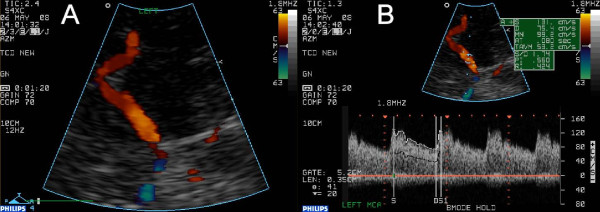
Ultrasound findings six weeks later. The aliasing in the most proximal segment is not present anymore despite a more sensitive setting of the colour scale. Accordingly, the Doppler spectogram shows completely normal blood flow velocities.
